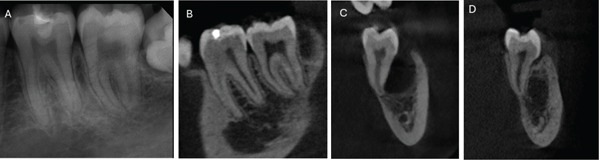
Preoperative CBCT and periapical radiograph and CBCT images at 1‐year follow‐up. (A) A postoperative periapical radiograph and (B) CBCT revealed an intact lamina dura with no signs of periapical radiolucency or pathology related to Tooth #18. (C) Preoperative CBCT coronal view shows an expansile nature of the lesion on the buccal surface. (D) Postoperative CBCT coronal view shows complete resolution of the cystic process and re‐establishment of buccal cortical and cancellous bone.
